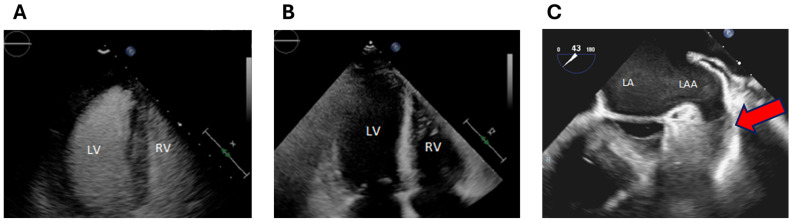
Imaging with Echocardiography. Panels (A,B) are transthoracic echocardiogram (TTE) apical four chamber views with and without ultrasound enhancing agent, respectively, showing borderline increased left ventricular wall thickness. Panel (C) is a transesophageal echocardiogram image demonstrating left atrial appendage with thrombus (red arrow) and spontaneous echo contrast in the left atrium. Abbreviations: LV—left ventricle, RV—right ventricle, LA—left atrium, LAA—left atrial appendage.
